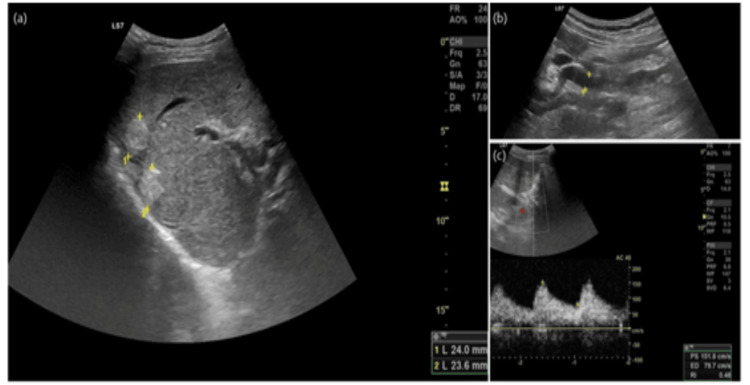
Abdominal ultrasound (a) In liver segment VII, hyperechogenic nodular lesions are identified, without vascularization in the color Doppler study. They translate to bile “lakes”/bilomas (with echogenic biliary debris) resulting from ischemia and biliary necrosis due to hemodynamic changes conditioned by arteriovenous malformations (AVMs); (b) dilated hepatic artery measuring 10 mm in caliber; (c) demonstrating increased velocities (PS: peak systolic velocity > 150 cm/s) and reduced RI: resistance index (0.48) in the Doppler spectral study, hemodynamic alterations resulting from the arteriovenous shunts.
